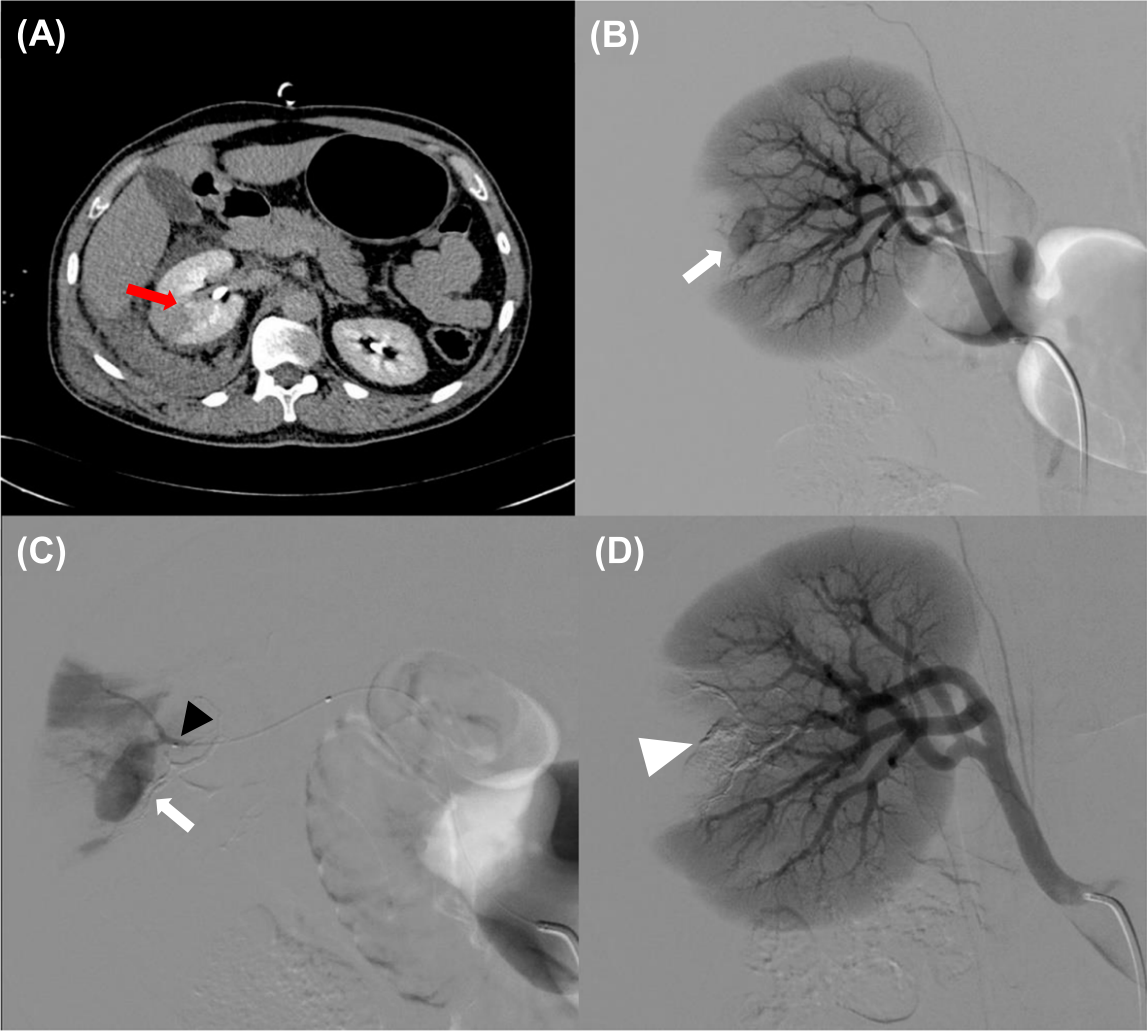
(a) Computed tomography (CT) image after endovenous contrast injection, depicting contrast deposition inside the right kidney. (b) Digital subtraction angiography (DSA) with injection from right renal artery showing pseudoaneurysm (white arrow), best seen in image (c) with a DSA performed by a microcatheter (black arrow) in the segmentar renal artery. (d) After embolization, glue cast (white arrow heads) in a subtracted image in the renal territory after embolization with complete superseletiva exclusion of the injured vessel from the circulation
